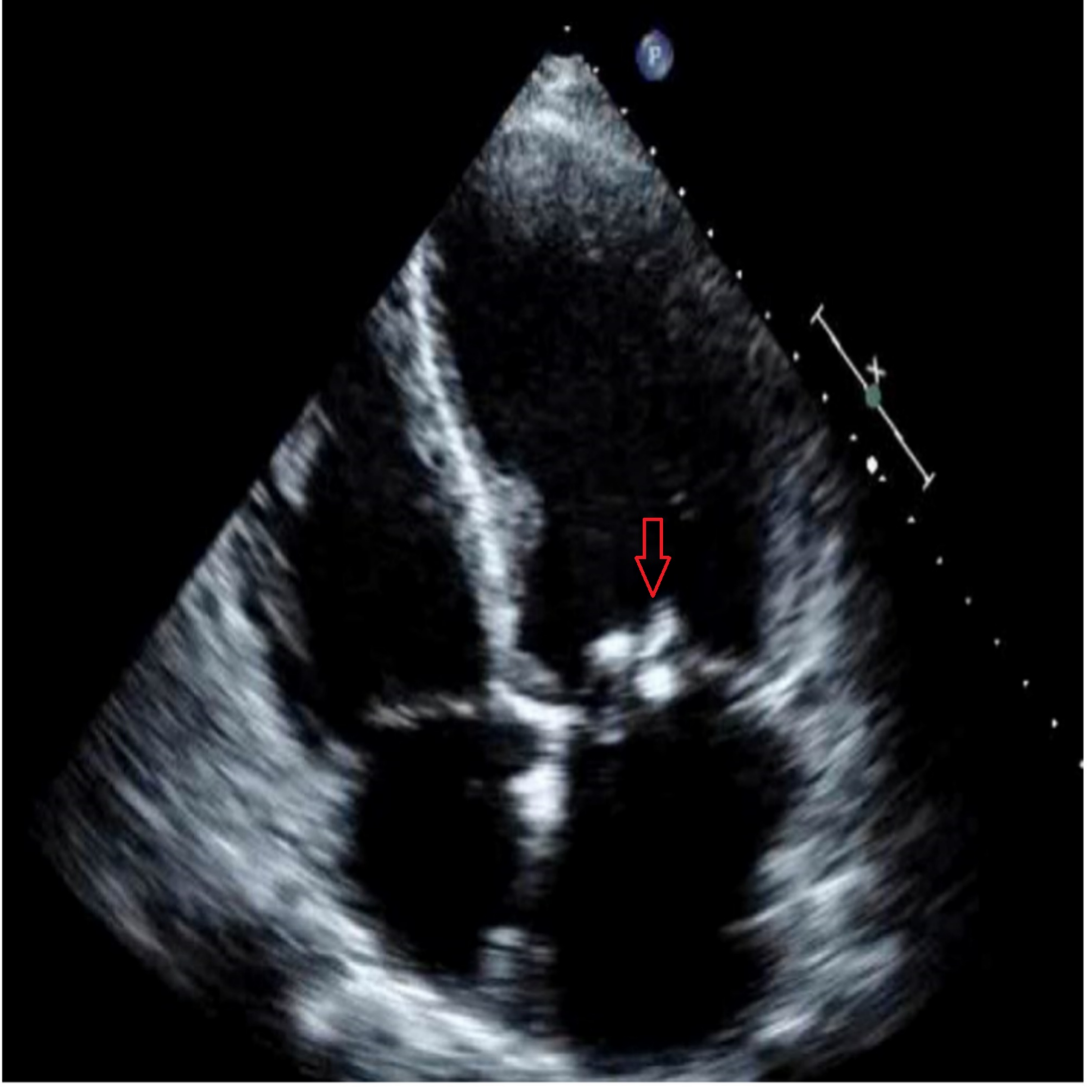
Transthoracic echocardiogram showing vegetations on mitral valve.
